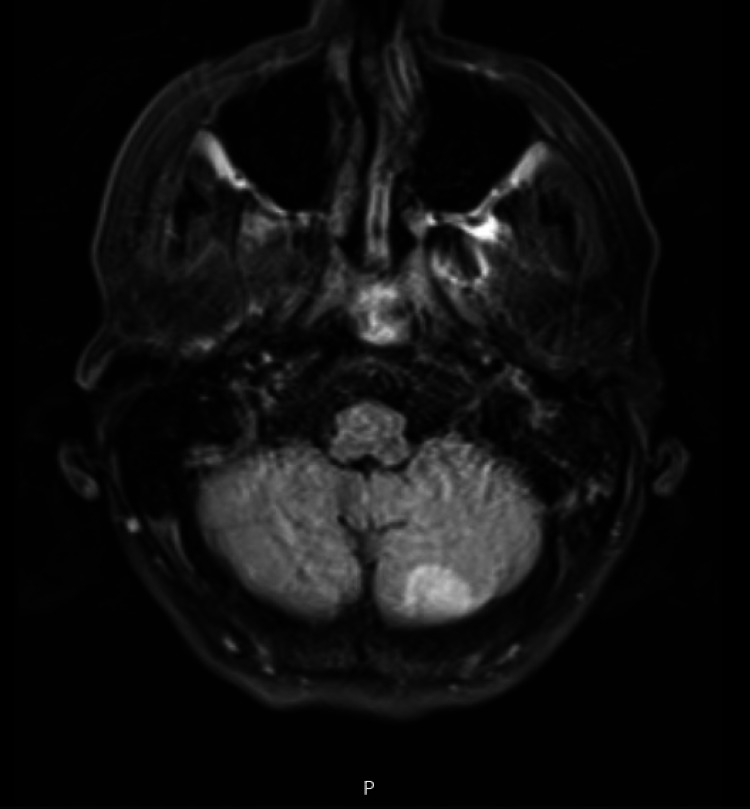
MRI brain showing left cerebellum diffusion abnormality consistent with acute ischemia.
